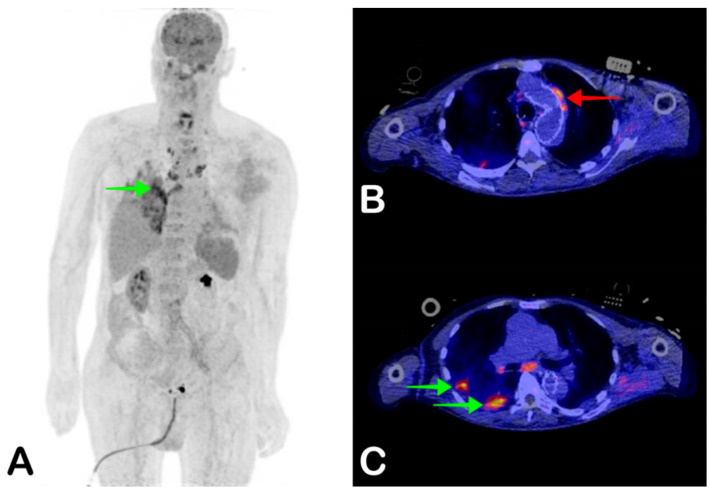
A 72-year-old man with a vascular graft in the aortic arch, abdominal aorta, and left common iliac artery was admitted with a fever of 38.5 degrees Celsius. Blood cultures were positive for *Enterococcus faecalis*. A clinical diagnosis of endocarditis was made based on the modified Duke criteria, although transthoracic and transesophageal ultrasound did not show signs of endocarditis. Coronal maximum-intensity projection FDG-PET showed increased FDG uptake in the right lower pulmonary lobe suggestive of infection (A, green arrow), and multiple metabolically active mediastinal and paratracheal lymph nodes. Fused axial FDG-PET/CT showed increased FDG uptake of the aortic arch stent suggestive of infection (B, red arrow), as well as FDG avid lesions suggestive of pulmonary infection (C, green arrows). The patient died three days after the PET/CT.
